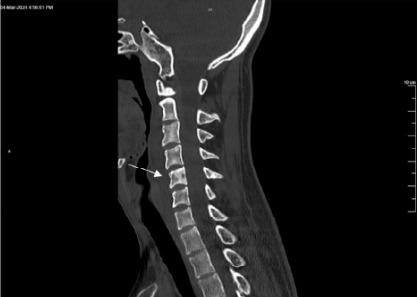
Sagittal computed tomography of the cervical spine without contrast demonstrating lucent expansile lesion involving the fifth cervical vertebral body (arrow), consistent with Ewing sarcoma.
